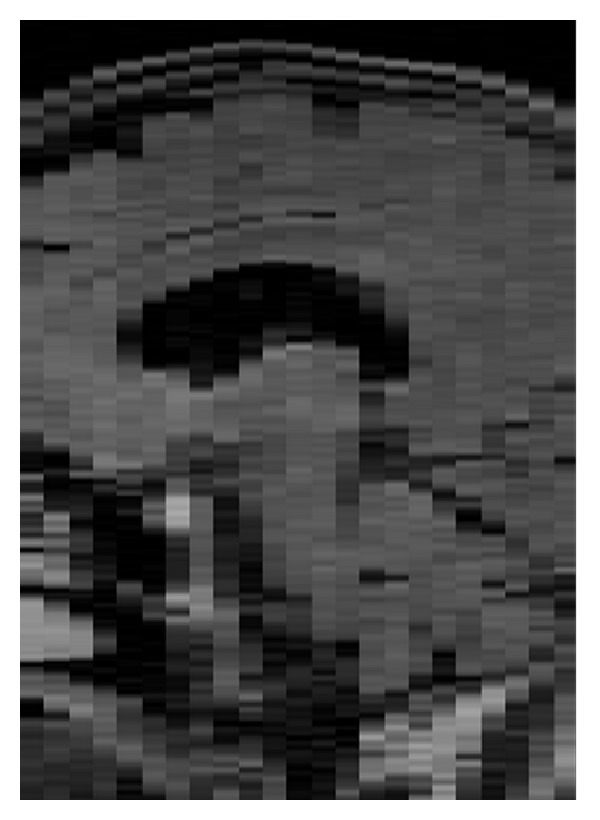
Sagittally reformatted slice from an example subject showing the common anterior intensity shift between the fourth and fifth slices in the coronal 2D FLAIR sequence.
